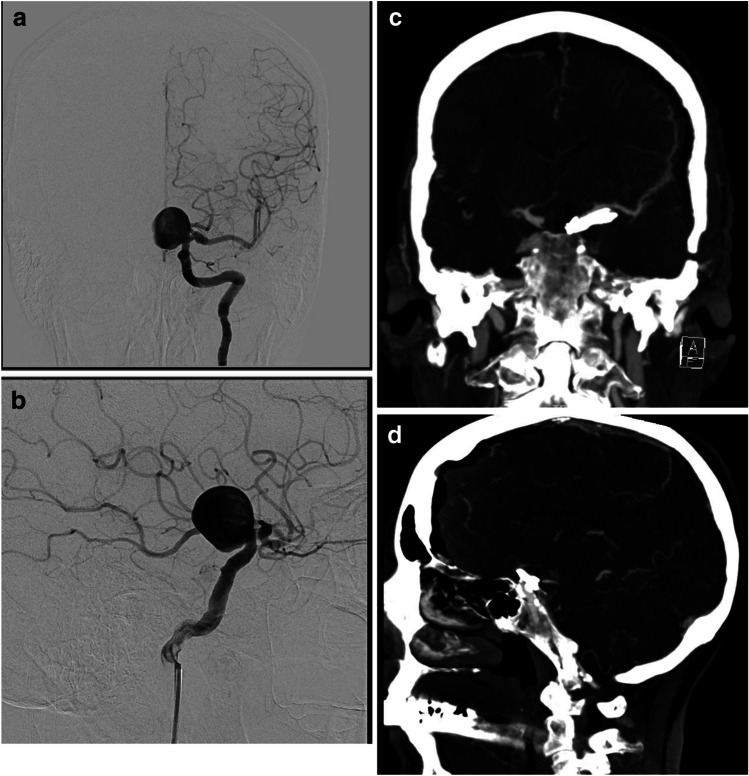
Pre- and post-operative imaging studies. A Pre-operative DSA, AP view, revealing a very large paraclinoid aneurysm. B Pre-operative DSA, lateral view, showing the very large paraclinoid aneurysm and the PCom aneurysm. C Post-operative CTA, coronal view: Complete obliteration of the aneurysm and filling of all blood vessels. D Post-operative CTA, sagittal view. Complete obliteration of the aneurysm. Please observe the calcifications inside the carotid at the level of the cavernous sinus and the supraclinoid segment
